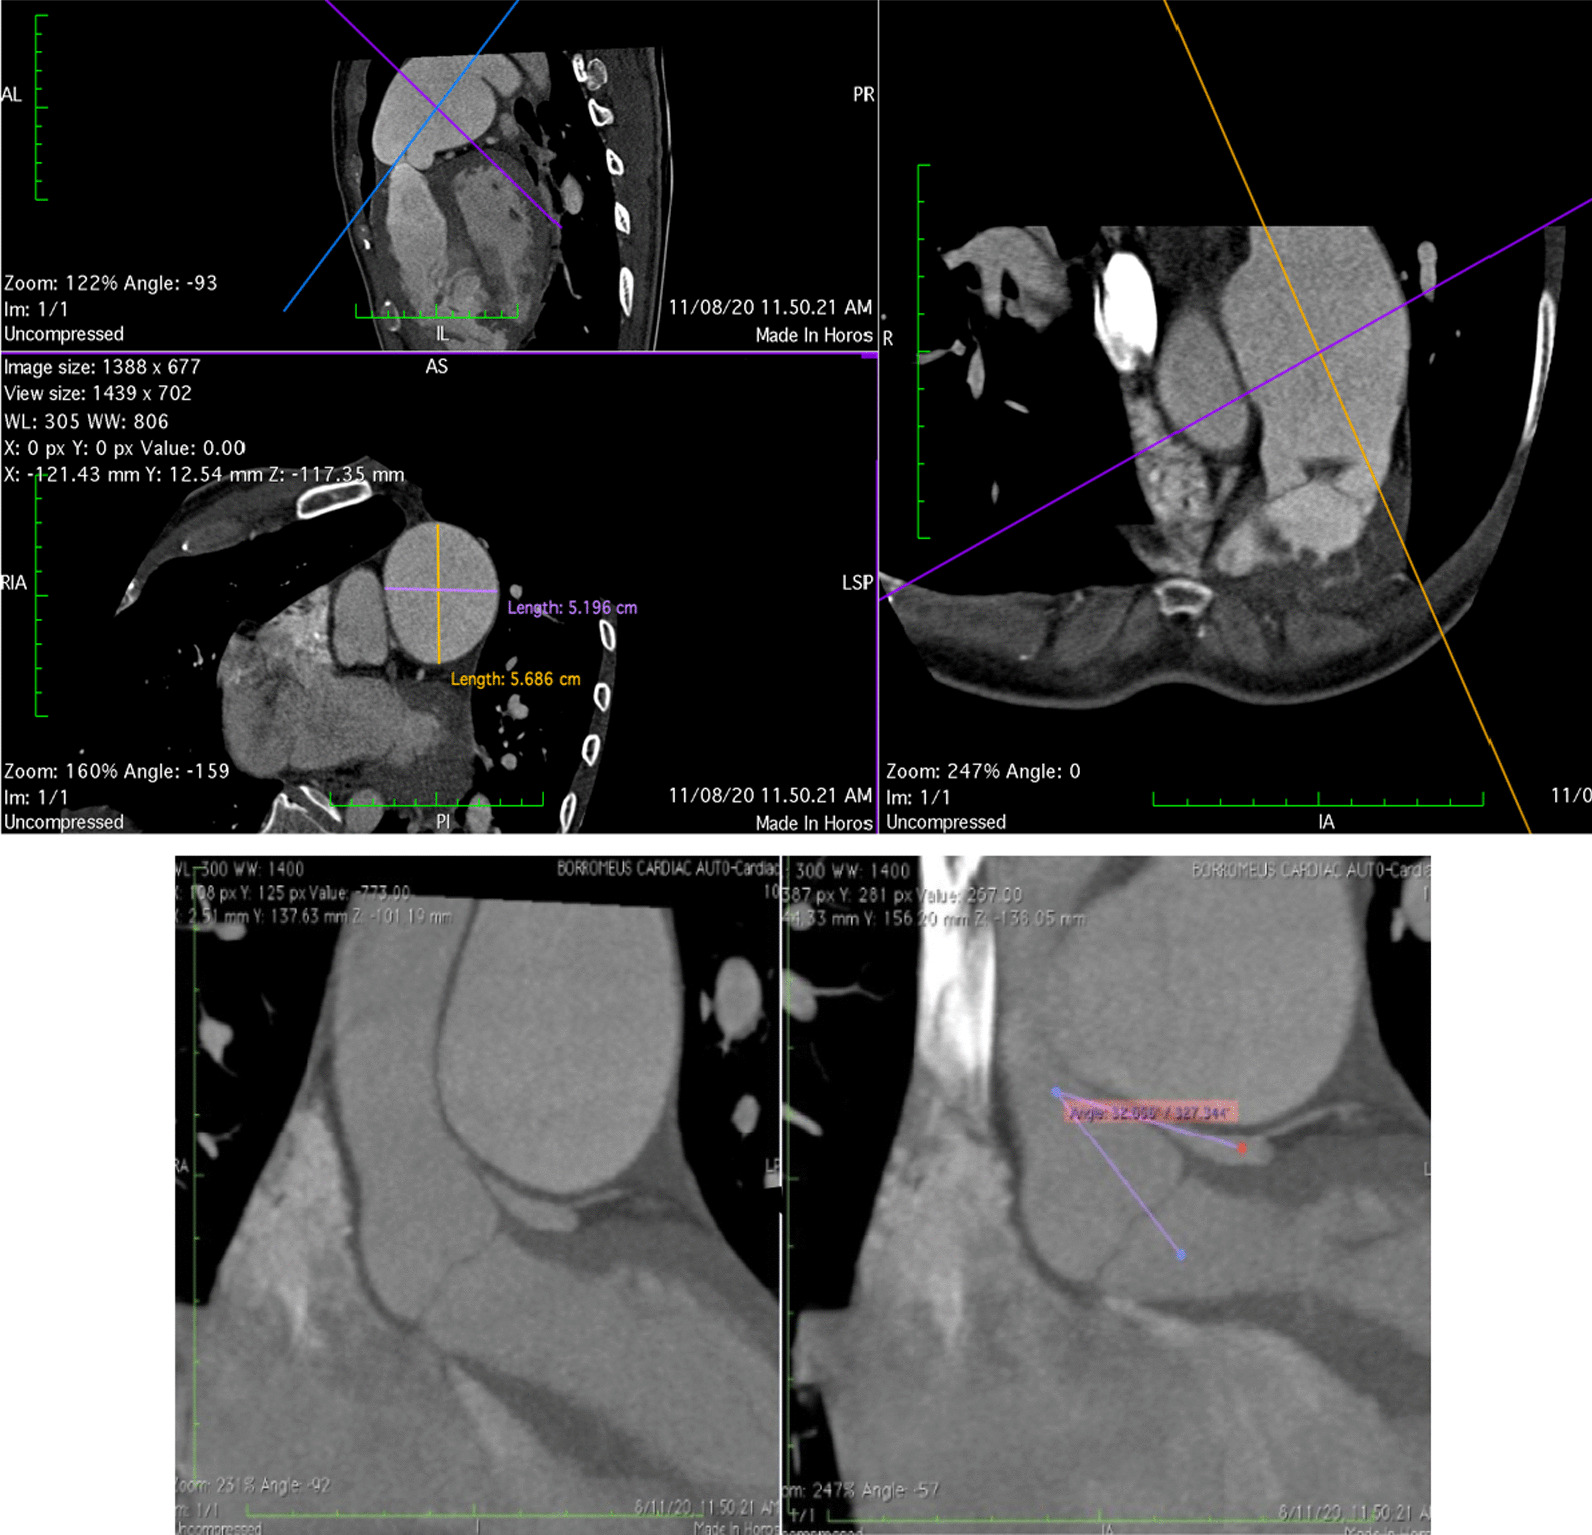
Contrast-enhanced cardiac CT images, (upper) images showed dilated MPA of 56.8 × 51.9 mm, (bottom left) black arrows showed severe compression of LMCA and (bottom right) LM take-off angle of 32°
